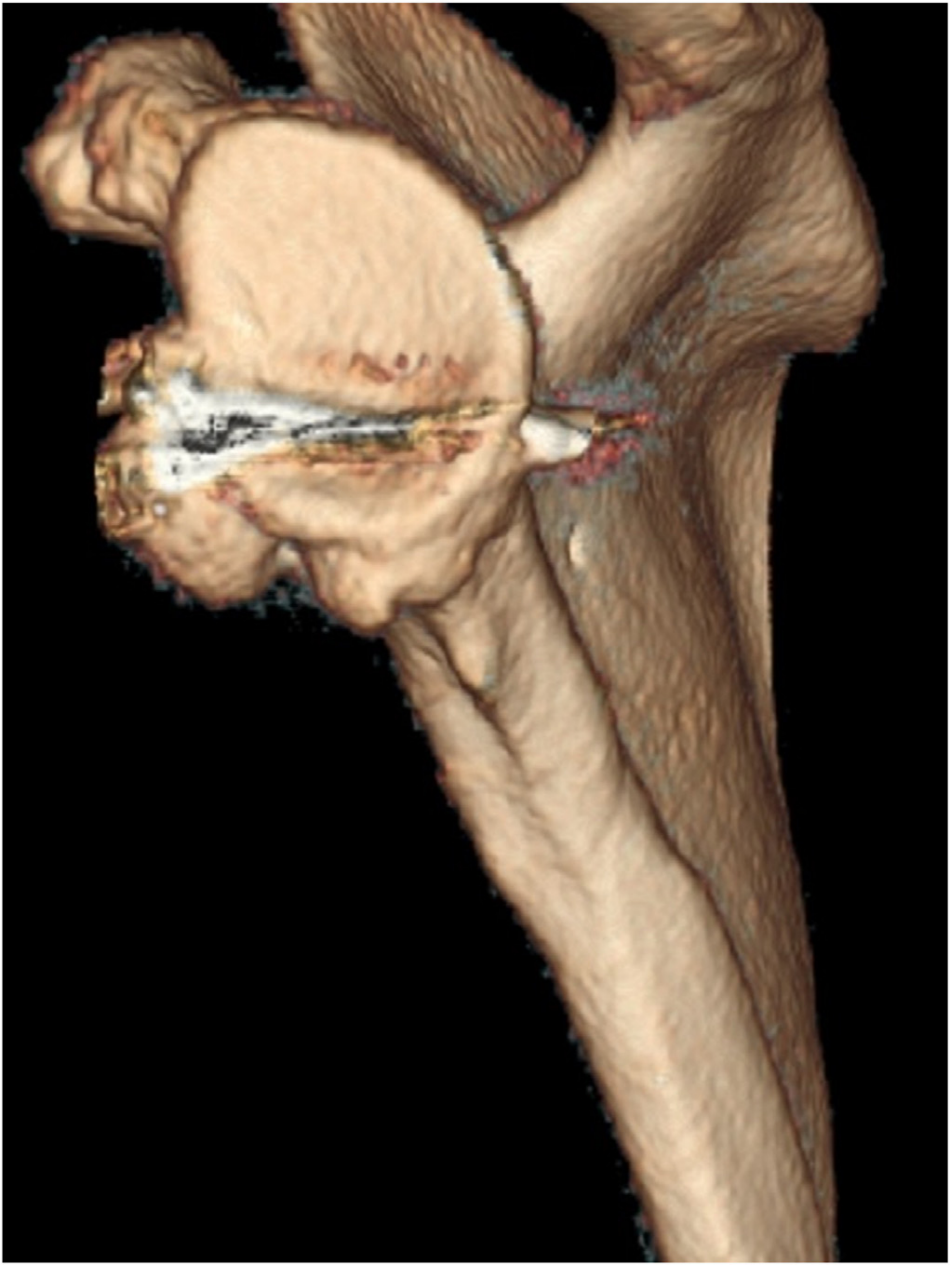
Three-dimensional CT scan image taken after posterior dislocation of lateral scapula and glenoid.
